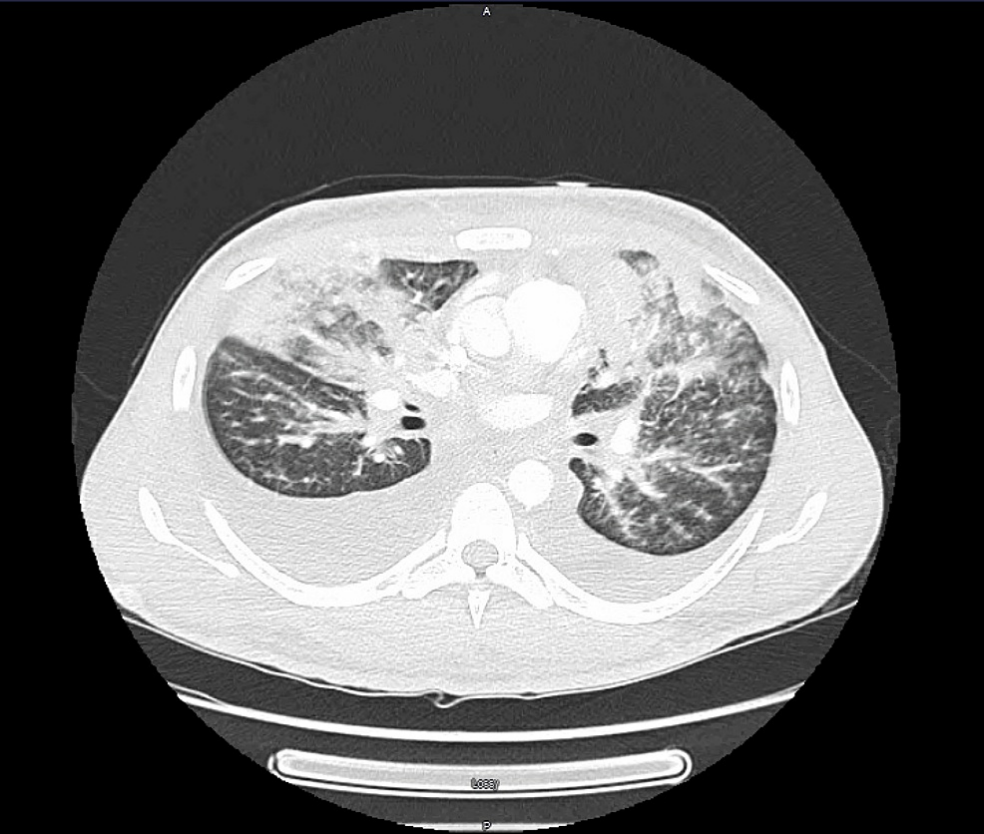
Cross-section of patient's chest CT showing ground glass opacities, air space opacities, and bilateral pleural effusions.
